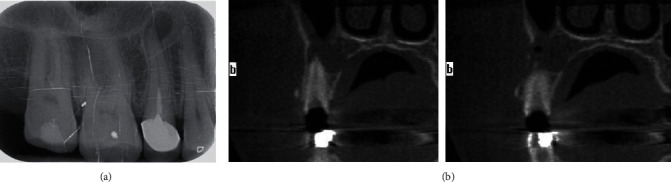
(a) Intraoral radiograph showed radiolucency in relation to the apex of the upper right second premolar. (b) CBCT radiograph showed a radiolucent image related to the apical part of #15 with bone resorption, discontinuity of the buccal and maxillary sinus cortical.
